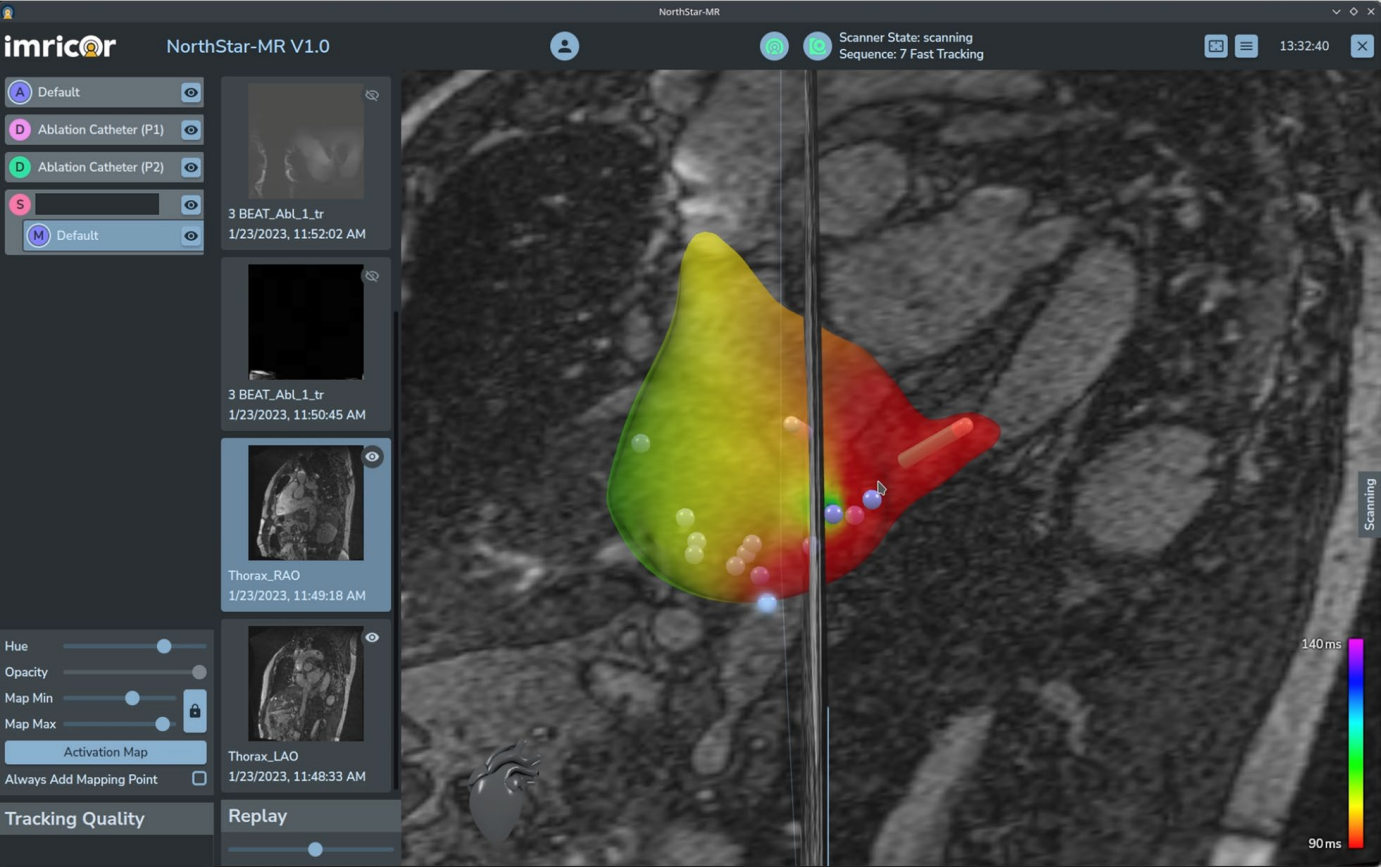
iCMR atrial flutter ablation. Activation map superimposed on anatomic MR images during CMR-guided atrial flutter ablation. Image courtesy of Ivo van der Bilt, MD and the MRI Ablation Center at Haga Teaching Hospital, Netherlands. CMR, cardiovascular magnetic resonance, iCMR, interventional cardiovascular magnetic resonance
